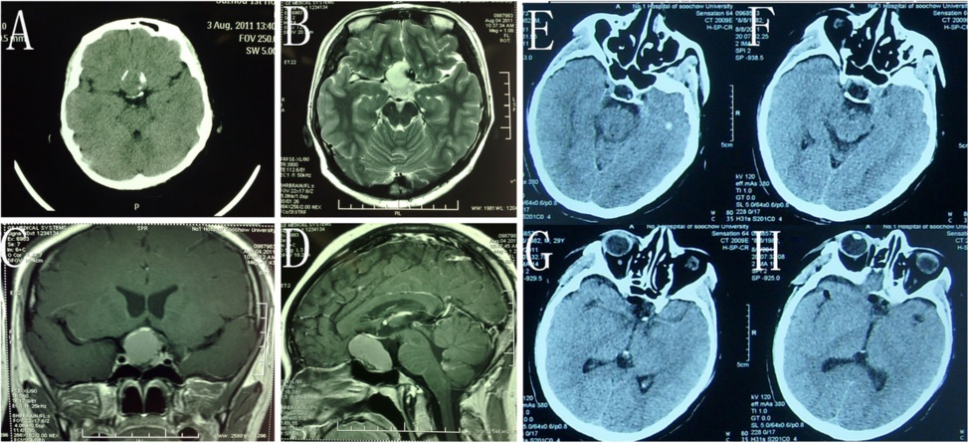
Case number 12: (A) preoperative CT imaging showing the calcification of craniopharyngioma; (B-D) contrasted magnetic resonance imaging showing a craniopharyngioma; (E-H) postoperative CT imaging of the same patient.
